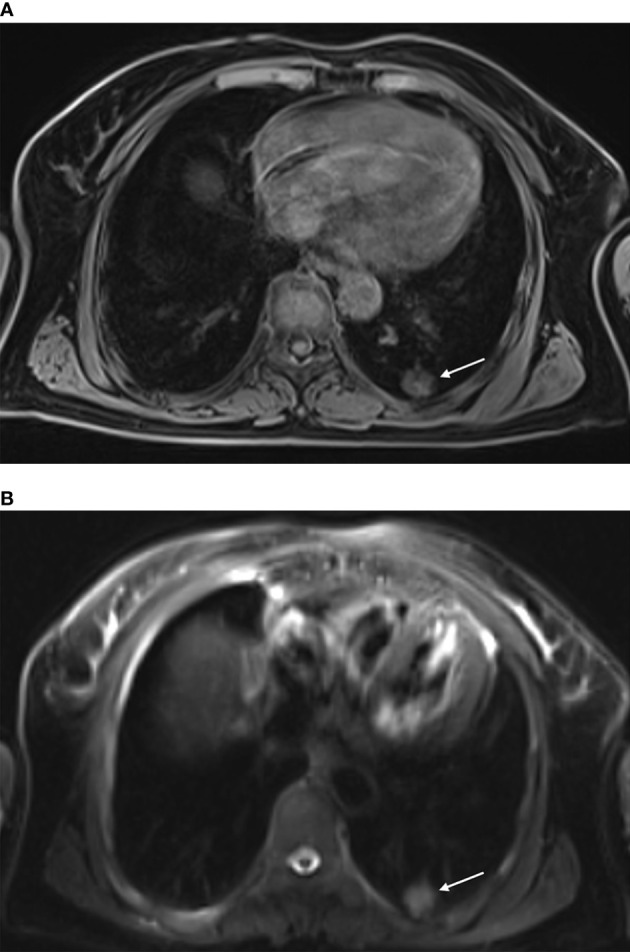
MR-guided MWA for invasive adenocarcinoma of the left lower lobe for 72-year-old woman. Preoperative scan: Preoperative MRI showed a nodule in the left lower lobe, displayed as isointense signals on T1WI [(A) arrow] and high signals on fsFRFSE T2WI [(B) arrow].
